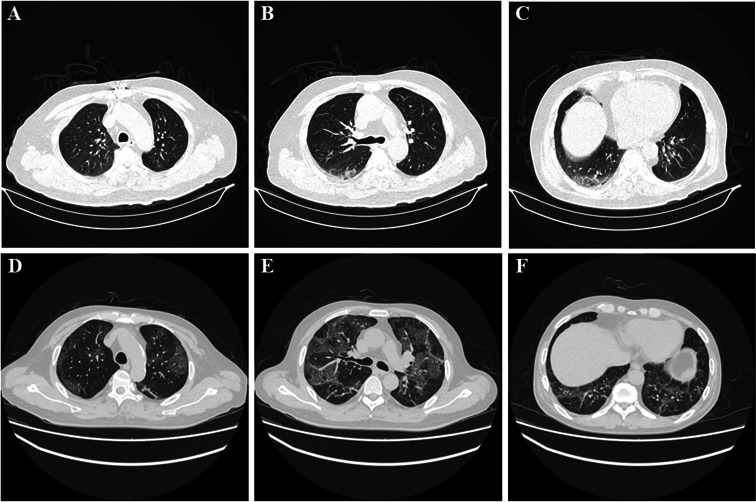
Chest CT image of APs with Omicron BA.1 infection on admission. (A–C) Chest CT scan of non-hypertensive patient, showing small patchy ground glass opacity in the left upper lobe and the right lower lobe; (D–F) chest CT scan of hypertensive patient, showing multiple patchy ground glass opacity and crazy-paving pattern in both lungs.
